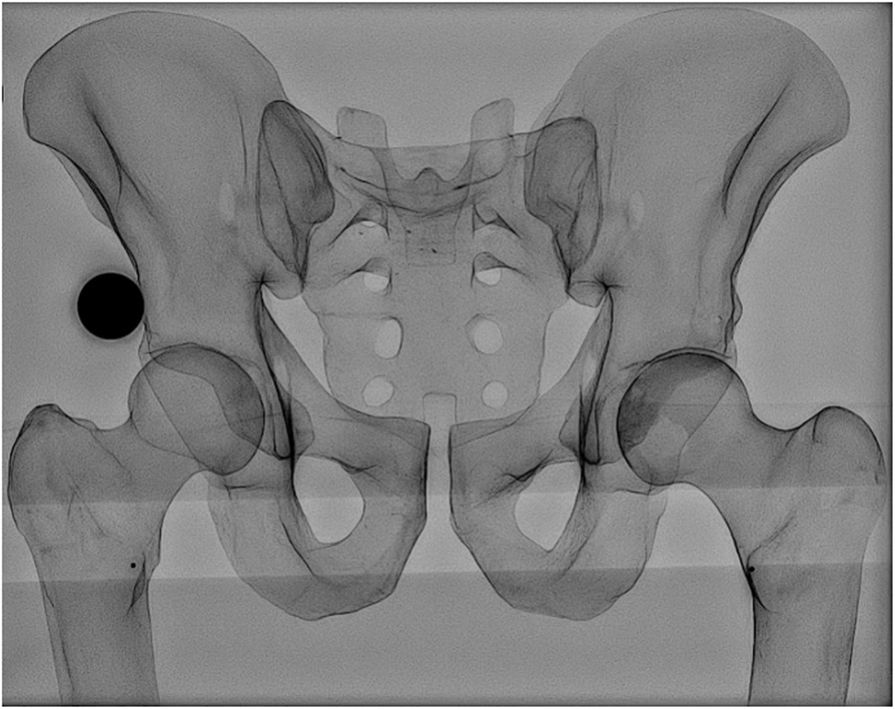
Anteroposterior (AP) radiograph of the Sawbones model, demonstrating its radiopaque cortical shell. For calibration and reference, a 25-mm steel ball is placed at the level of the acetabulofemoral joint and a 2-mm metallic marker at the lesser trochanter
